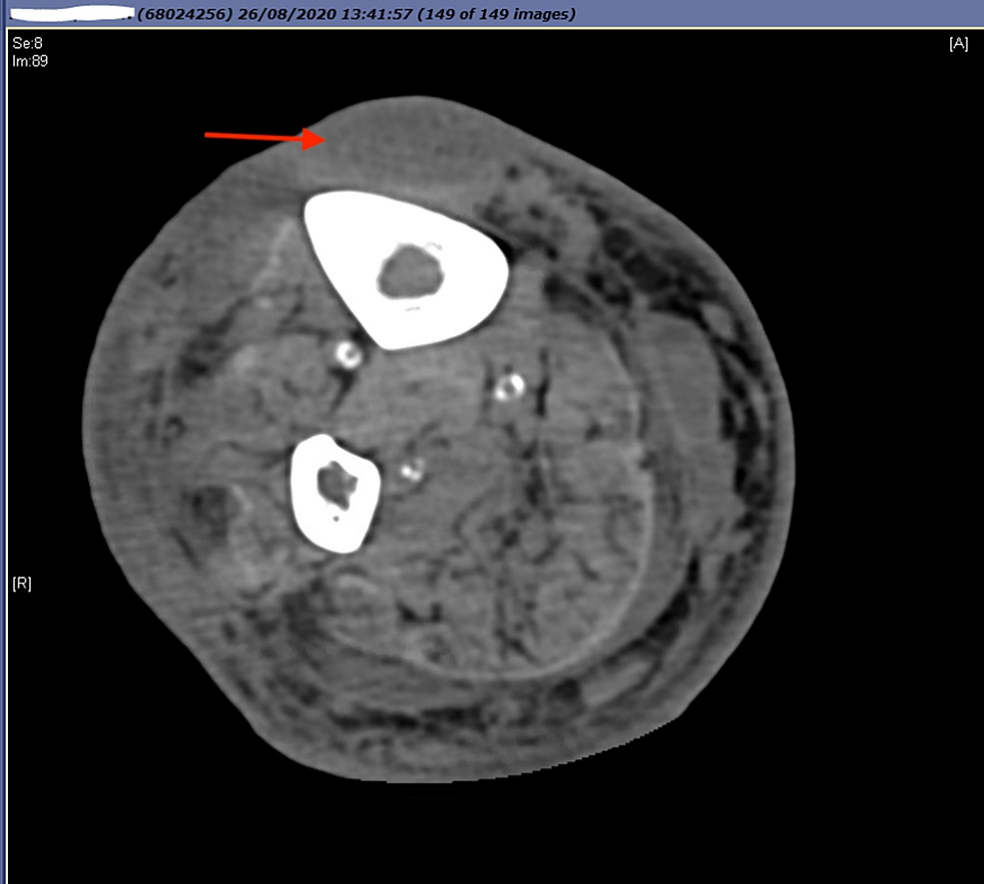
Axial CT scan of the right tibia + fibula demonstrating an anterior tibial collection (red arrow).
